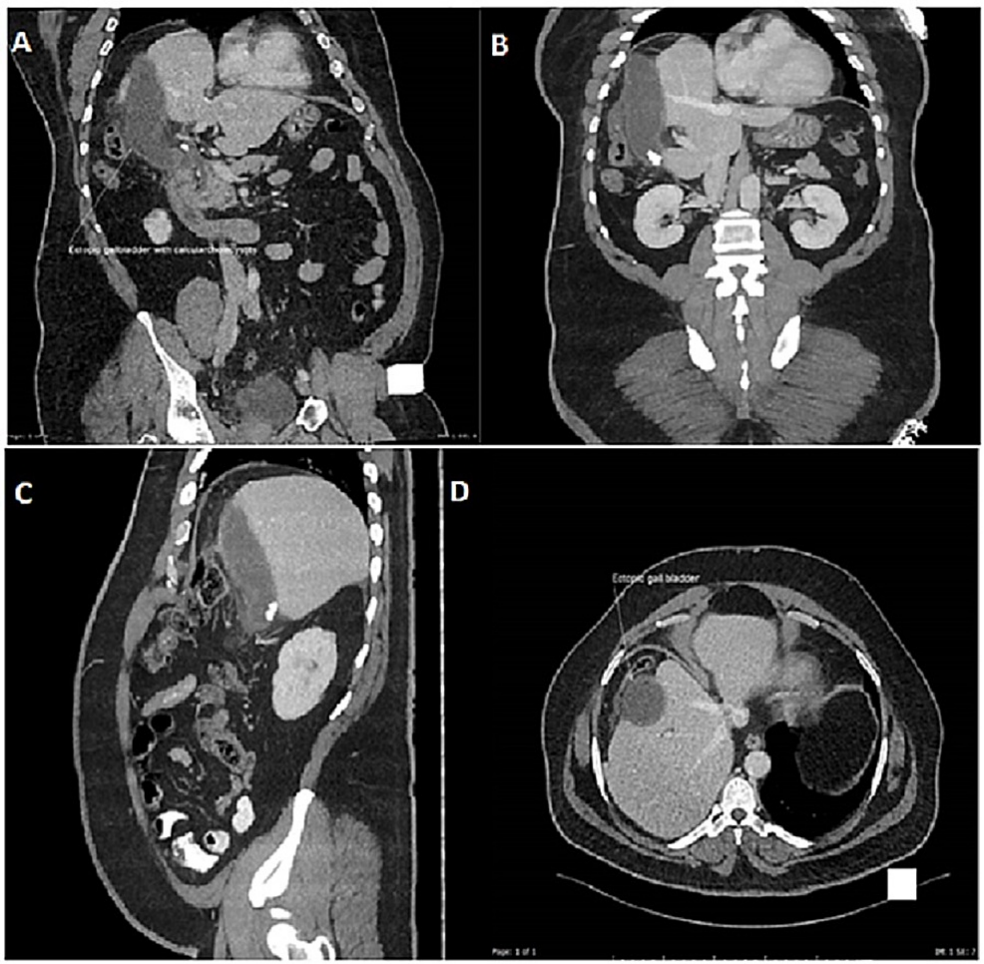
Contrast-enhanced CT scan of the abdomen in axial (A, B), sagittal (C), and coronal (D) views demonstrating the unusual location of gallbladder located lateral to the right lobe of liver with associated features of acute calculous cholecystitis. Partial hypoplasia of the right liver lobe and mild eventration of the right hemidiaphragm is also demonstrated.
